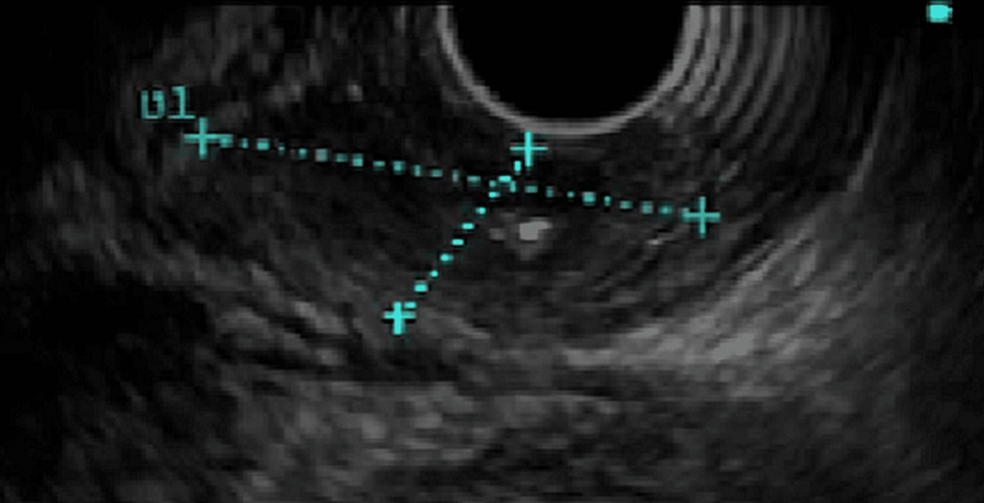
EUS of the esophageal lesion (blue dashed lines) EUS: endoscopic ultrasound.
